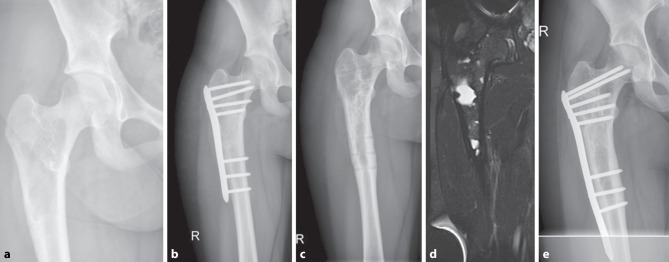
A 12-year-old patient with a proximal femoral UBC (a) received curettage, burring, filling with allograft bone chips and plate fixation in surgery. (b) Material removal surgery was performed 20 months afterwards (c) and  5 years later a progressive recurrent osteolysis was observed in follow-up MRI (d), which led to revision curettage, burring, filling with allograft bone chips and plate fixation (e)
